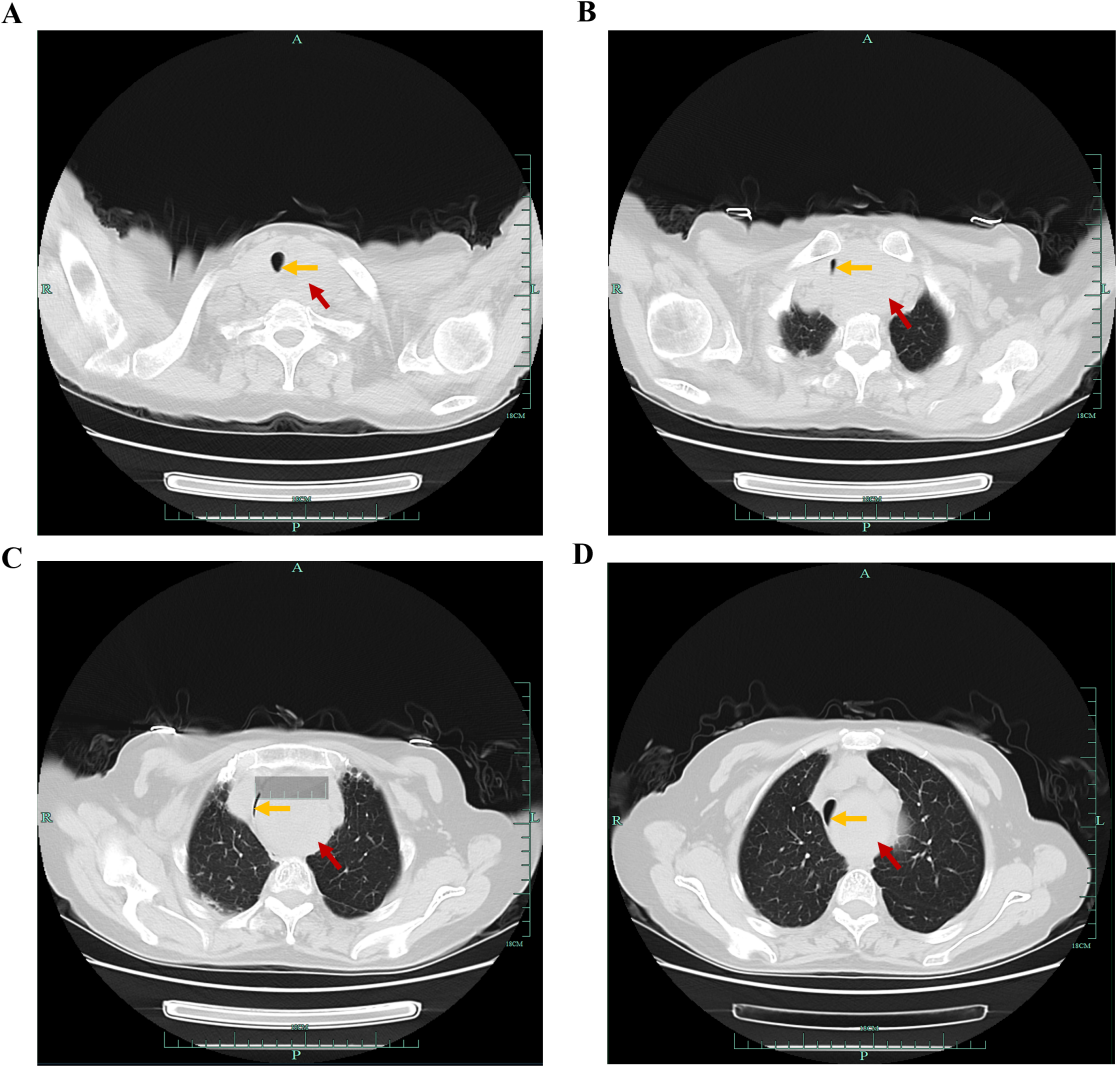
Preoperative computed tomography (CT) imaging of the head and neck. The CT scan of the neck and chest showing a large mass situated posterior to the left side of the sternum, causing substantial tracheal stenosis (A-D). The narrowest tracheal diameter in the supine position is approximately 2.0 mm (C). Red arrow is the mass; Yellow arrow is the narrowed tracheal compressed by the mass.
